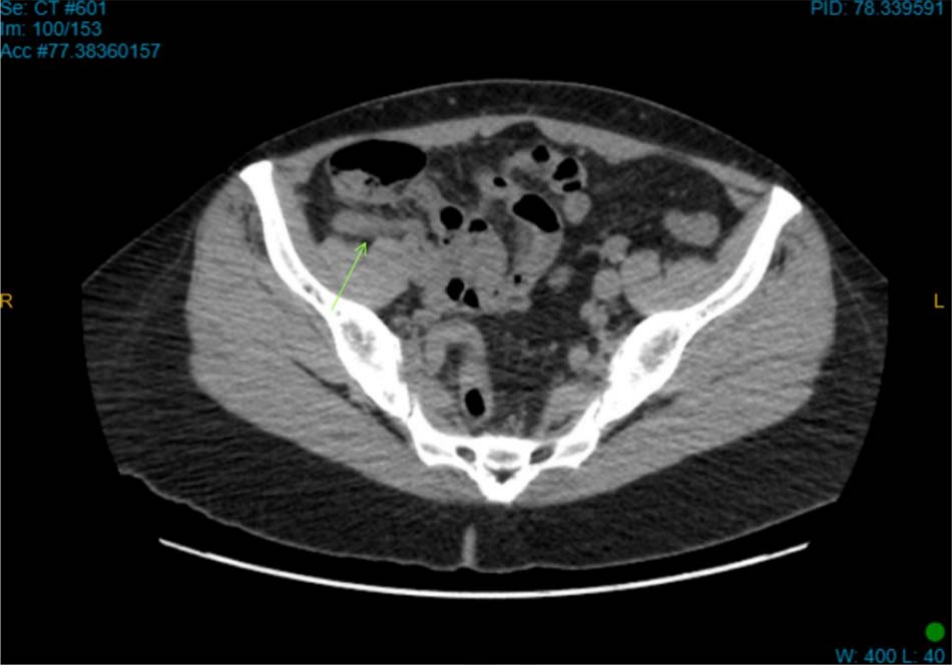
Axial CT image. Retro-cecal inflamed appendix.
